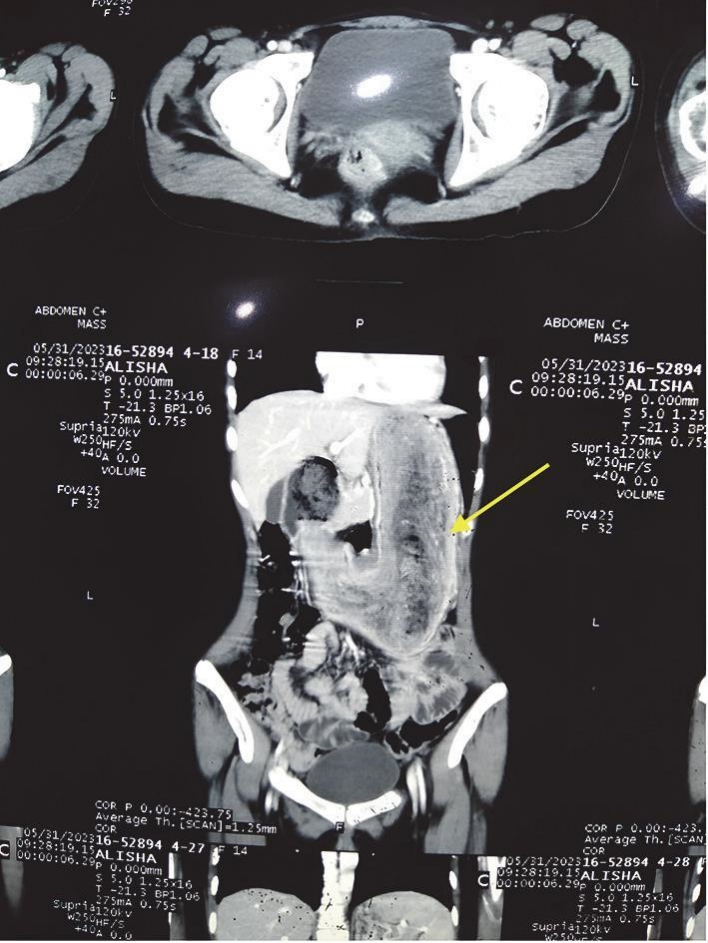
CT scan abdomen and pelvis of the patient. Large heterogeneous lesion (arrow) filling gastric lumen and duodenal bulb; incidental retroaortic left renal vein noted.
